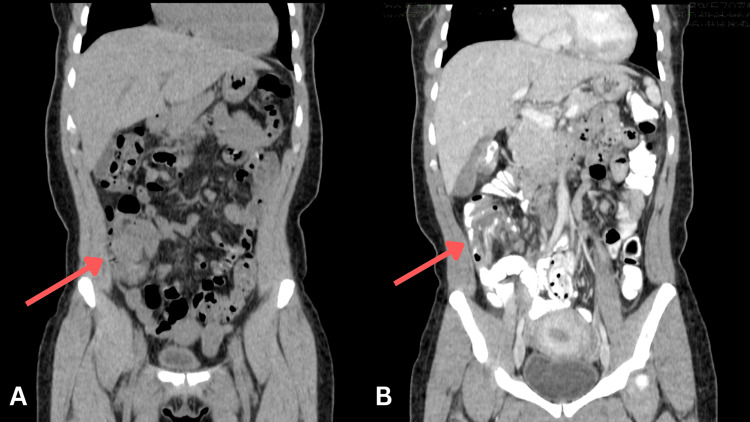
CT image of the abdomen and pelvis, coronal view, no contrast (A). CT image of the abdomen and pelvis, coronal view, with contrast (B). Red arrows point at ileocolic intussusception without contrast (A) and with contrast (B). CT: computed tomography
